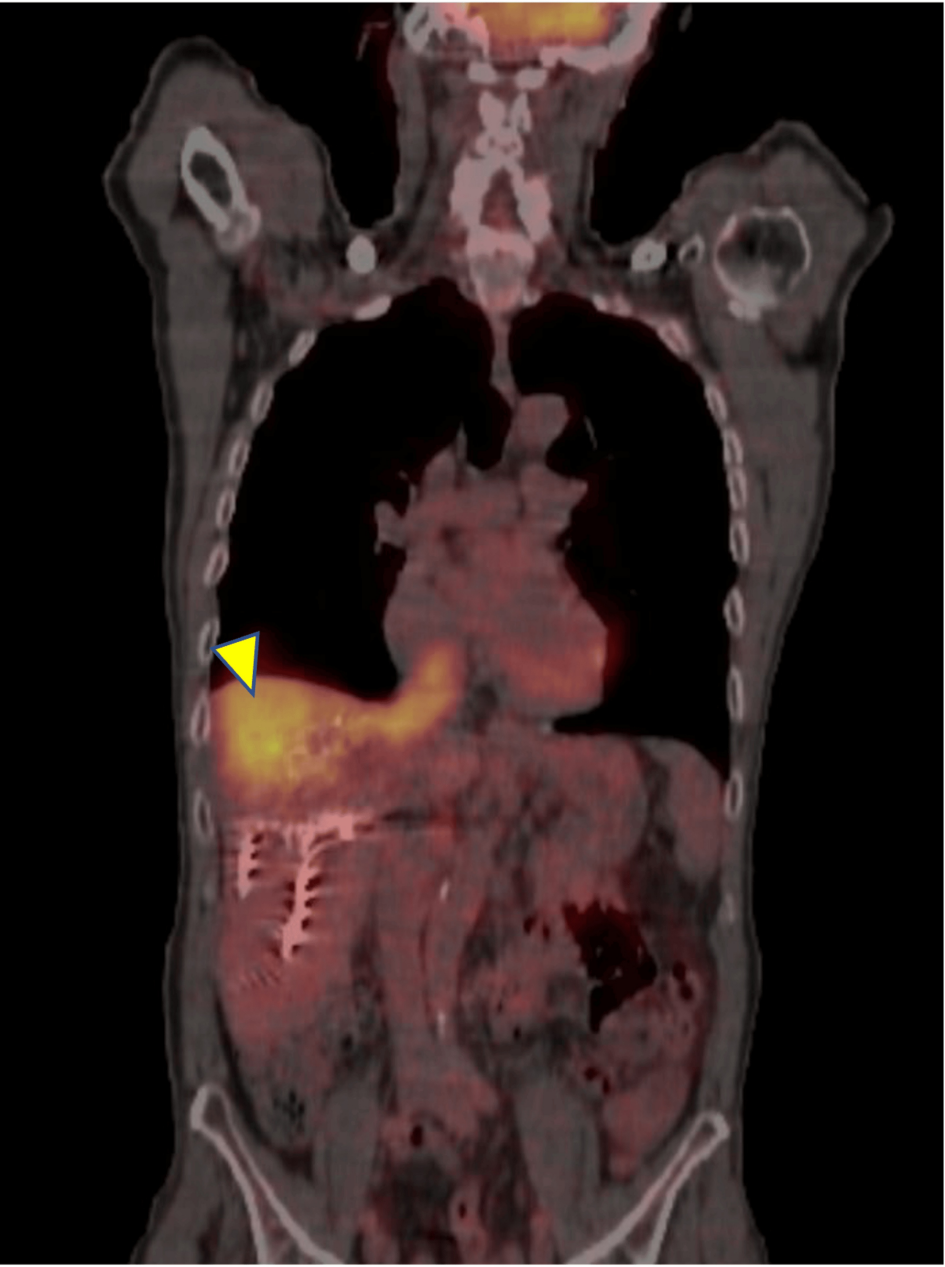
18F-FDG PET/CT imaging Coronal fused 18F-FDG PET/CT image demonstrating increased tracer uptake corresponding to a tumor thrombus extending from the right hepatic vein into the inferior vena cava (arrowhead). 18F-FDG PET/CT: fluorine-18 fluorodeoxyglucose positron emission tomography/computed tomography
